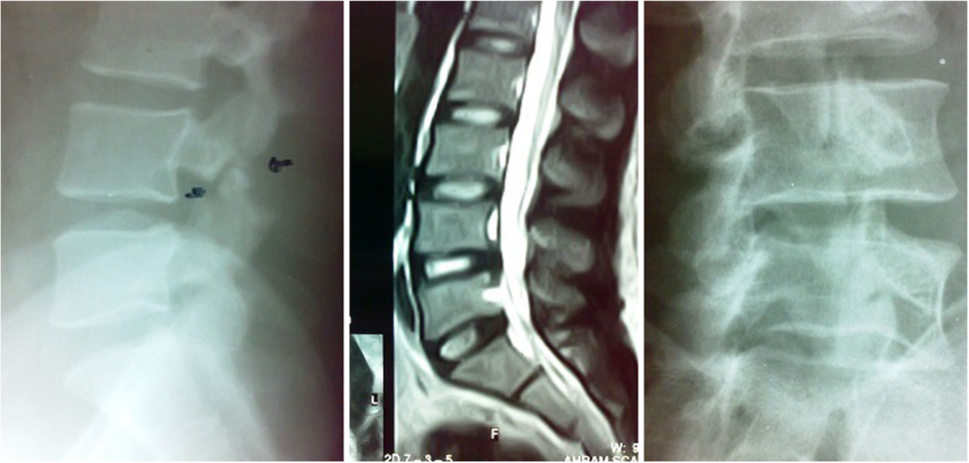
Computed tomography assessment of the union at three and six months (left, center) and postoperative lateral image of the construct (right) showing apparent bony union of the defect.
